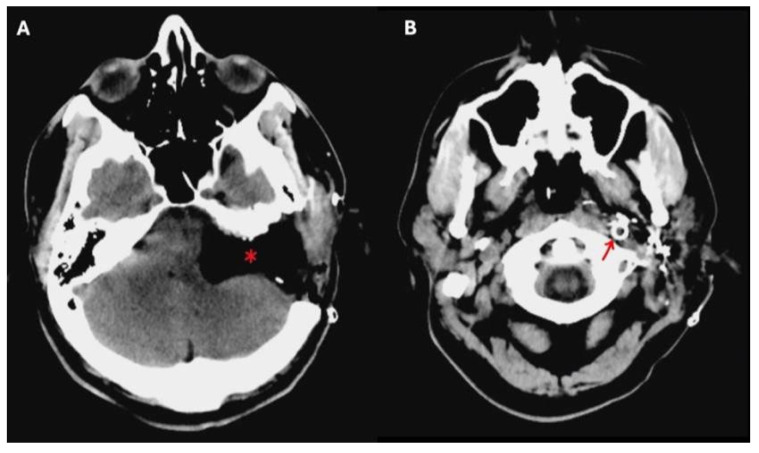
A postoperative brain CT scan after total removal of a TJ-PG Di2 in a single-stage procedure. In (A), * indicates the surgical cavity after the total removal of the TJ-PG Di2. In (B), the red arrow indicates the endovascular stent placed preoperatively in the internal carotid artery.
